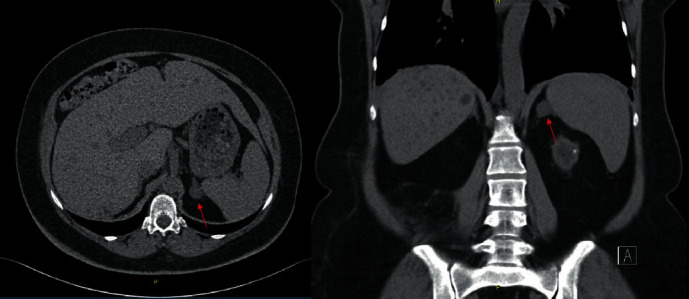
CT scan. Axial and coronal planes of CT scan showing left adrenal mass (indicated by red arrow).
